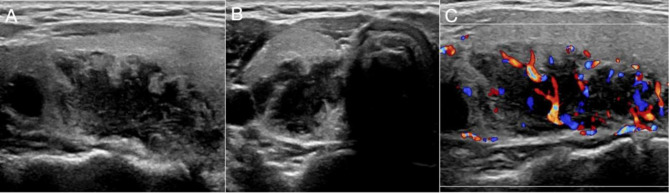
US images of a 9th week-pregnancy female, with pathological results showing an atypical parathyroid tumor. A.B. The longitudinal and cross section images showed an irregular shape and unclear boundary. C. Color Doppler US showed abundant blood flow signals
